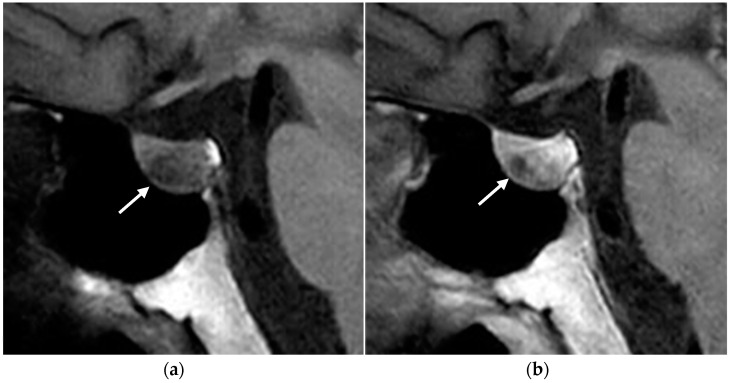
Pituitary micro-prolactinoma. T1-weighted MR images before (a) and after (b) contrast administration showing a hypointense and low-enhancing nodule (arrow) in the anterior pituitary gland.
